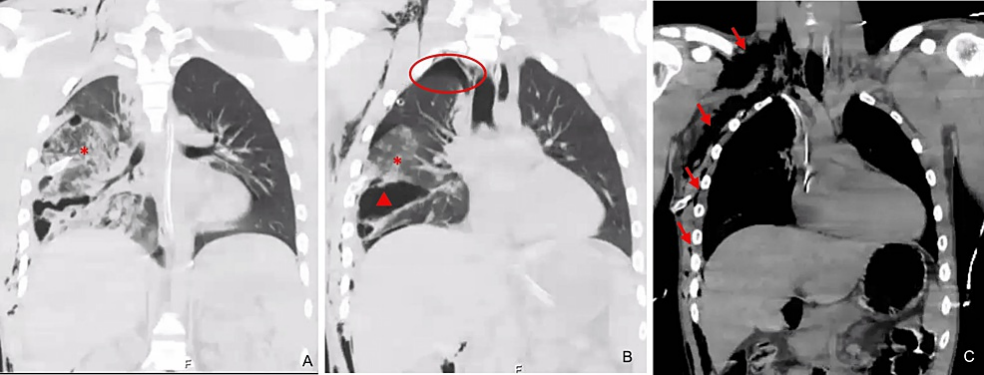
Coronal CT reconstructions from the lung (A, B) and mediastinal (C) windows revealing a right pneumothorax (circle), extensive pulmonary contusion (*), and laceration (triangle) of the right lung parenchyma. It also shows an extensive subcutaneous emphysema involving the right thoracic wall that extends to the neck (arrows).
